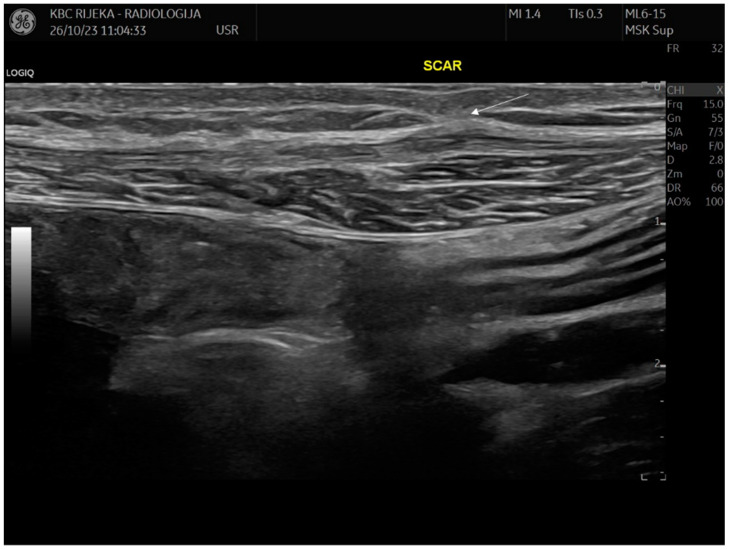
Anterior abdominal wall ultrasound examination. An ultrasound examination of the anterior abdominal wall with a linear 15 Hz probe 5 years after resection with minimal scarring (arrow) of the left rectal muscle without signs of recurrence.
